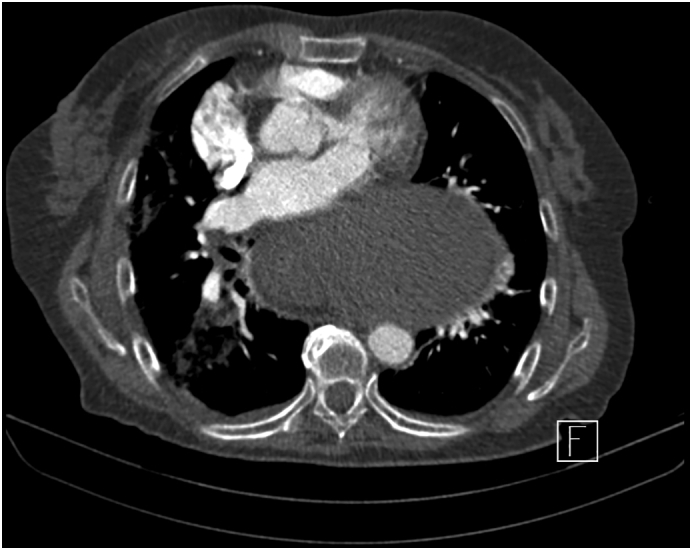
CT of mixed hiatal hernia - transverse section.
